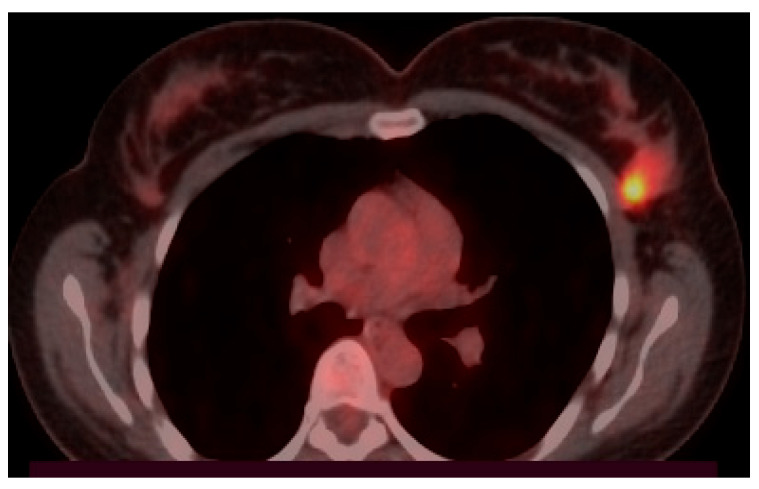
Metabolic active tumor on the left breast PET-CT aspect (institutional patient data).
